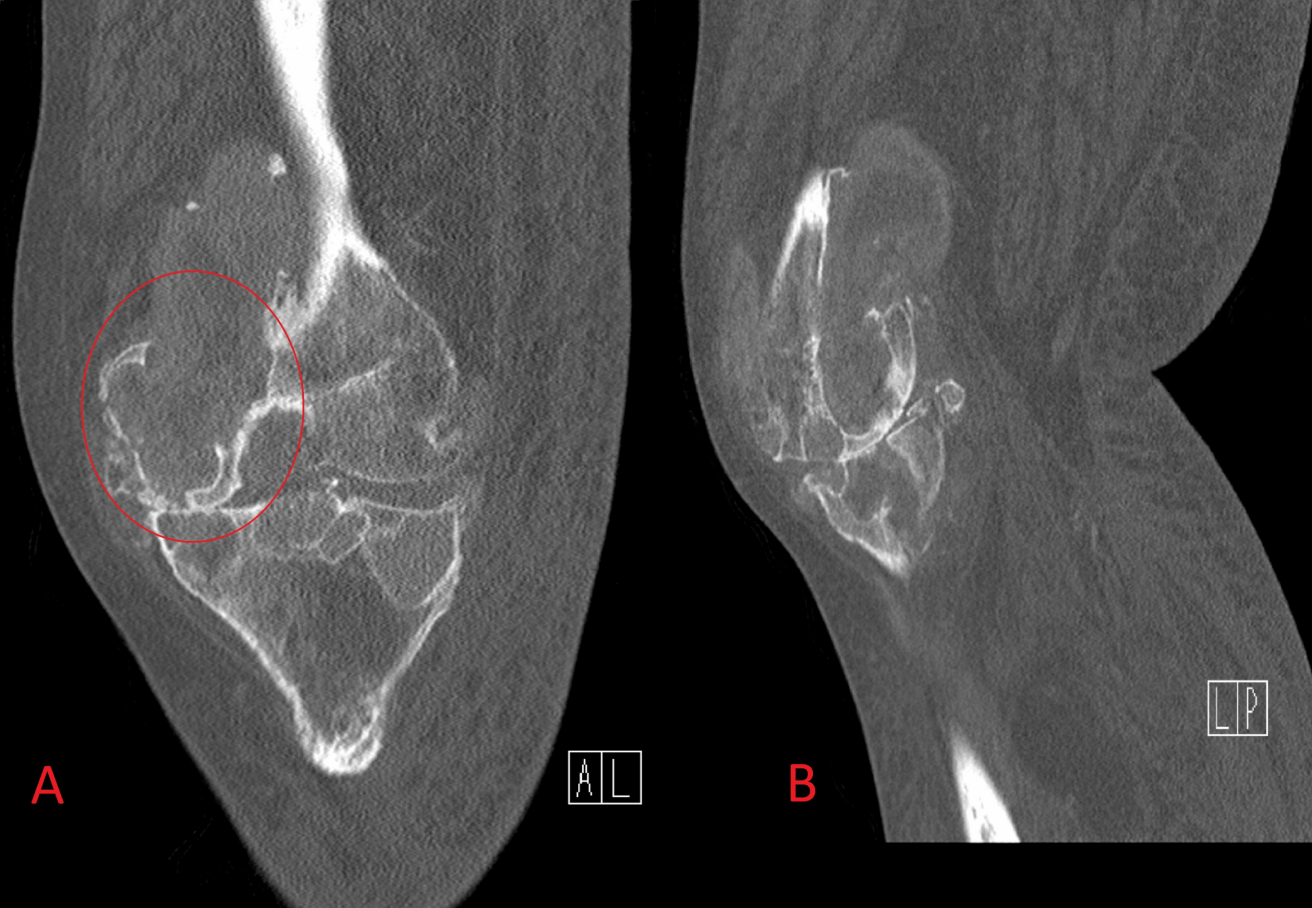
The CT scan demonstrates subcortical cysts with intra-articular soft tissue extensions and extensive cortical destruction with decreased joint space. 2A: coronal reconstructed image; 2B: sagittal reconstructed image
